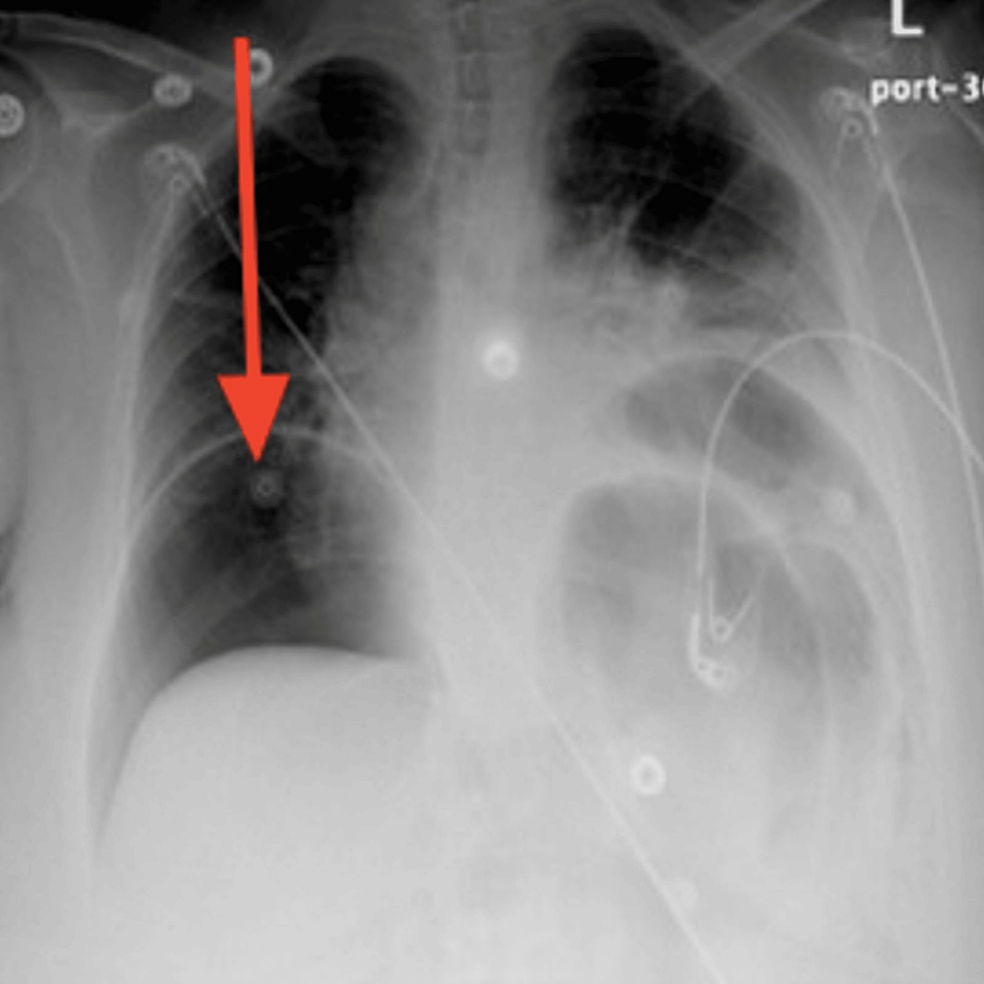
Posteroanterior chest X-ray at the time of gastric perforation displaying severe air under the diaphragm with bilateral obstruction indicating massive pneumoperitoneum (red arrow)
